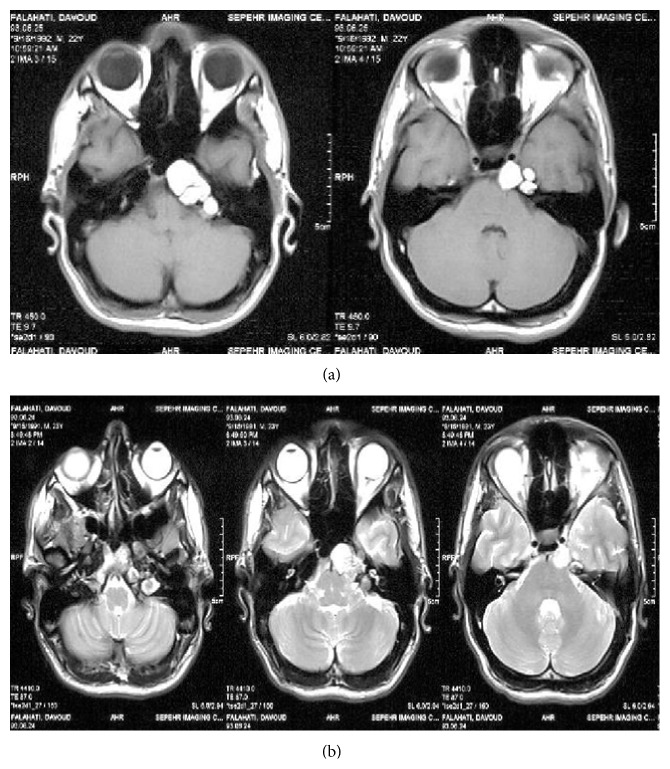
(a) T1 weighted MRI shows the cholesterol granuloma involving the right petrous apex. (b) Axial T2 weighted MRI shows the residual tumor at petrous apex adjacent to right carotid artery.
